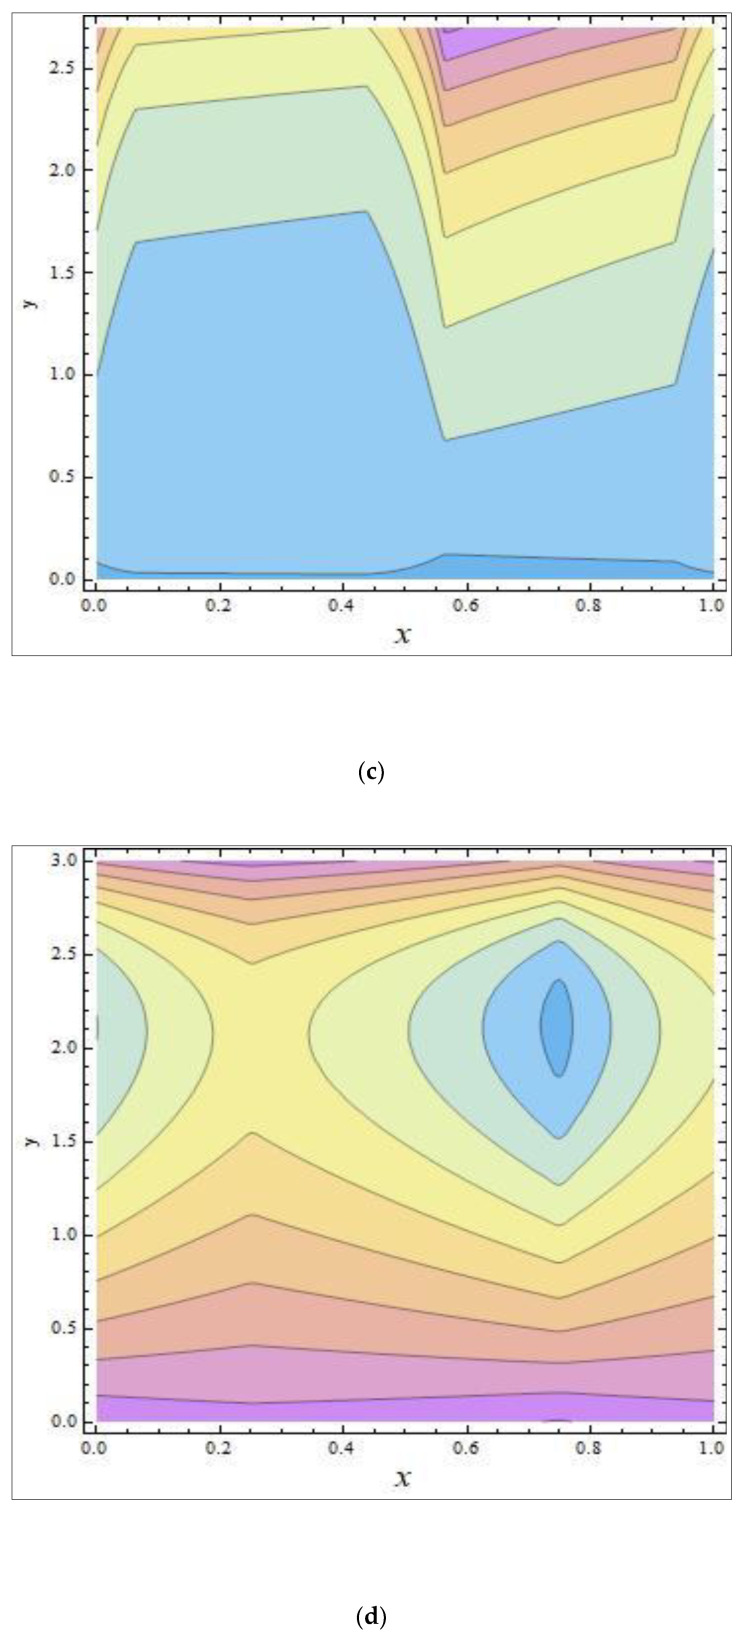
The streamlines of different wave shapes: (a) sinusoidal wave; (b) multi-sinusoidal wave; (c) trapezoidal wave; (d) triangular wave. The values of the other parameters were $N_{CT}=0.3,\;N_{TC}=0.7,\;G_{rc}=0.4,\;G_{rF}=0.1,\;G_{rt}=0.5,\;N_{t}=0.7,\;M=3.5,\;\phi =\frac{\pi }{6},\;\Gamma =0.8,\;\beta =0.7,\;m=0.6,\;Q=2.37$.
